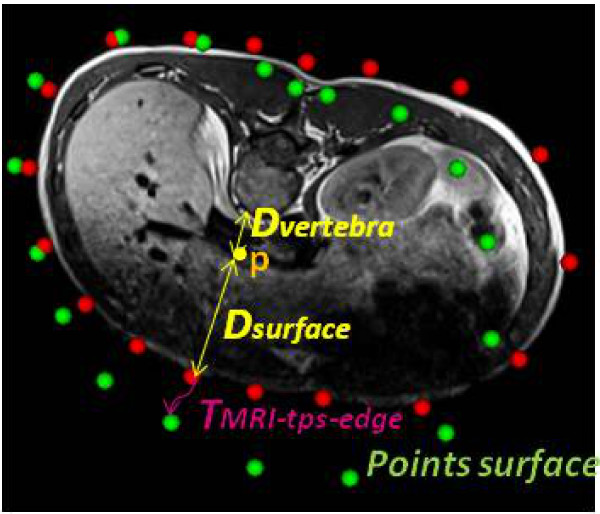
Ratio for weighted thin-plate spline. Calculation of the weighted thin-plate spline ratio based on the distance of the point *p* to be registed, the closest vertebra, and the surface of the torso. The points in green are obtained from the contours of the surface topography and the points in red are obtained from the MRI.
